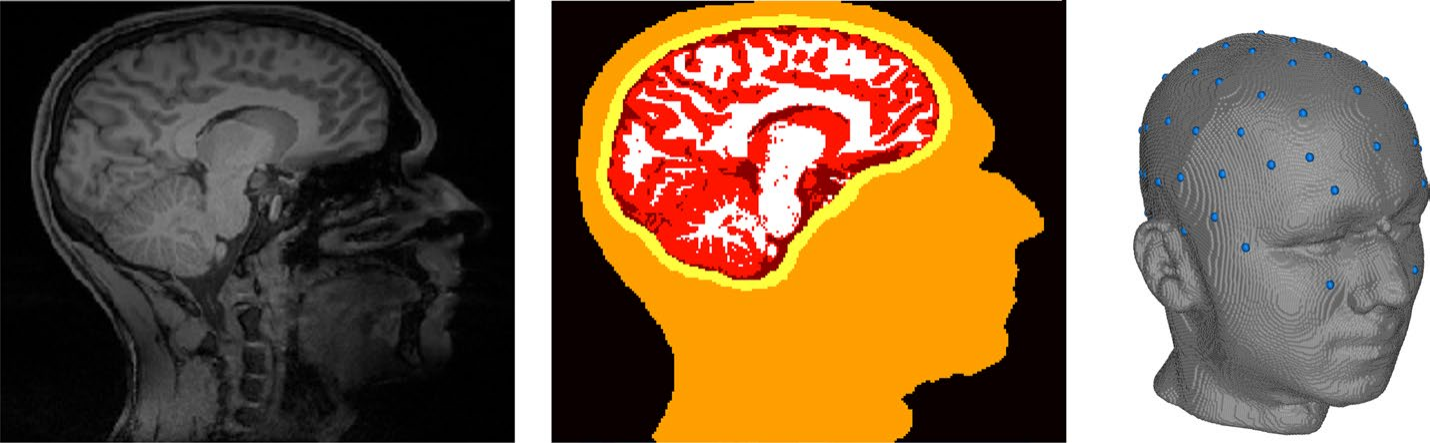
Original MRI (left), segmentation (middle), sagittal slice in T1-MRI space, and hexahedral mesh with aligned electrodes (right)
